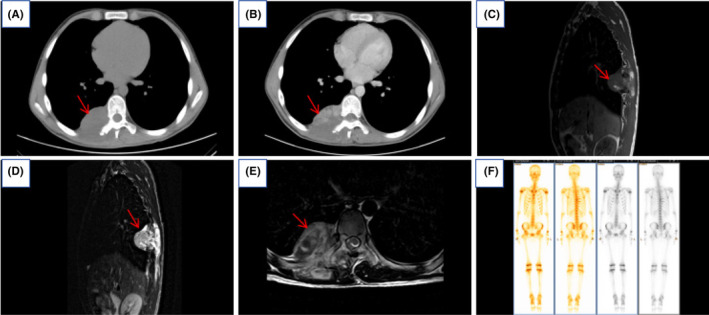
The enhanced scan of first computed tomography (CT) and magnetic resonance imaging (MRI) was admission: (A) The CT scan showed a right paravertebral mass at the level of the 8th and 9th thoracic vertebrae. (B) Enhanced CT scan showed marked inhomogeneous enhancement of the lesion, which is poorly demarcated from the right thoracic erector spinae muscle, with swelling and hypodensity of the right thoracic erector spinae muscle, and flocculent enhancement is seen within it (red arrows in A and B). MRI: (C) The T1WI sagittal lesion was isosignal with small patches of mixed high signal seen within it. (D) T2WI sagittal showed mixed signals with predominantly high signals. (E) T2WI cross‐sectional compression fat showed mixed signals of high and low with slightly high signals, and the lesion partially protruded into the spinal canal at the level of the thoracic 7 and 8 vertebrae, with compression of the spinal cord, and localized bone destruction of the right 8th and 9th posterior ribs and the right transverse process of the thoracic 8, with distended changes in the 9th posterior rib (red arrows in C, D and E). (F) Emission computed tomography (ECT) showed right 8 and 9 posterior ribs with localized concentration of bone nuclei and normal distribution of nuclei in the rest of the skeleton.
